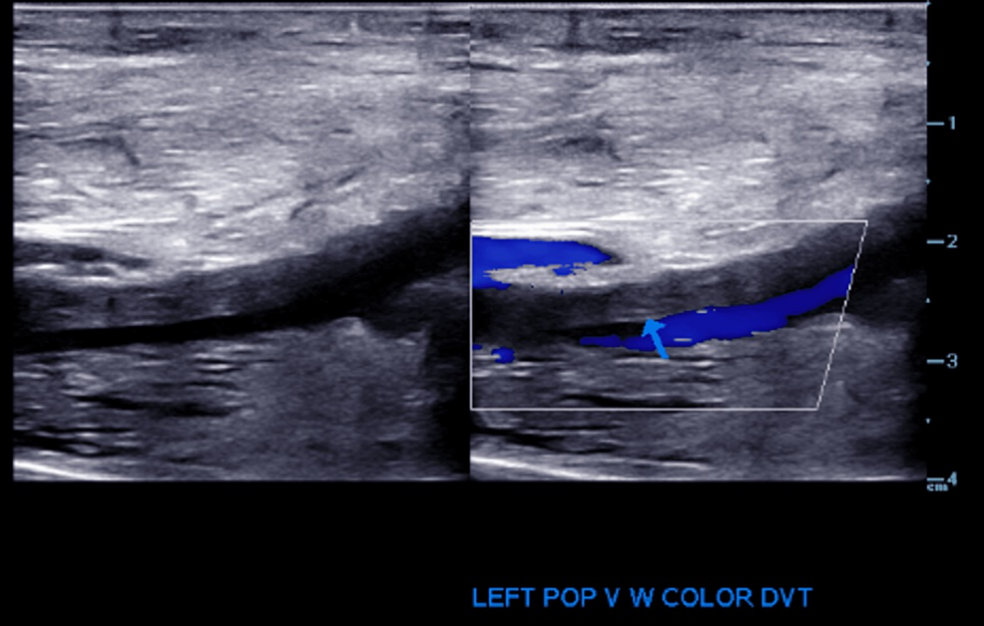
Venous duplex ultrasound: longitudinal view: left popliteal vein showing thrombus.
